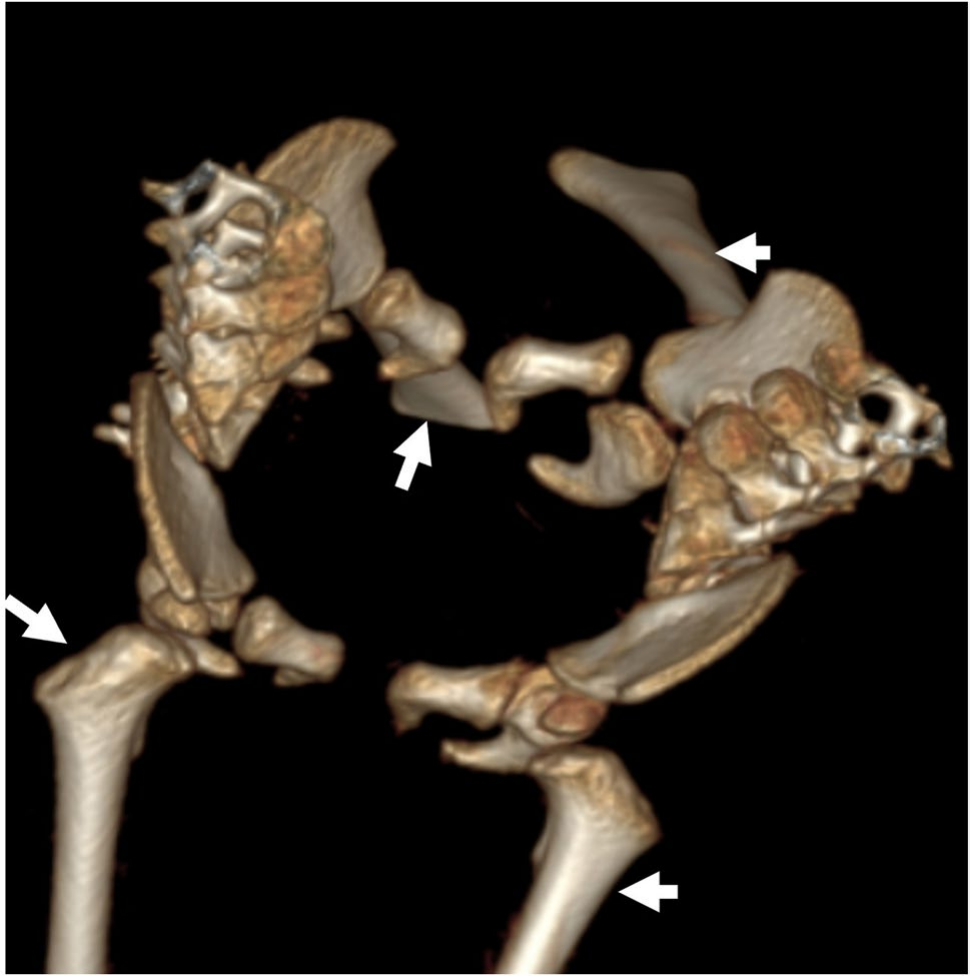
Pelvic fusion of 4-month-old ischiopagus tetrapus conjoined twin boys (same patients as in Figs. 7, 8 and 10). 3-dimensional computed tomography reconstruction of the bony pelvis shows that each baby has two normal lower limbs (*arrows*)
